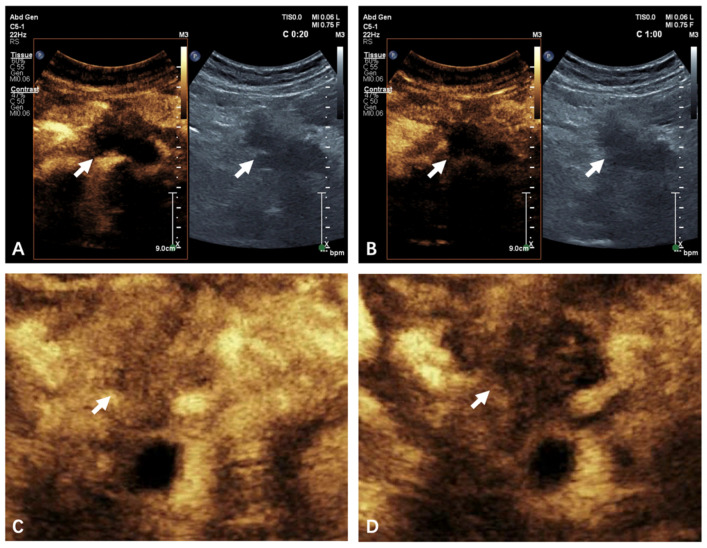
US+CEUS and CEUS-ROI images. (A,B) An example of typical US+CEUS frames of 20 s (arterial phase) (A) and 60 s (venous phase) (B) of a patient with ineffective treatment outcome, showing hypo-enhancement pattern. (C,D) An example of typical CEUS-ROI frame of 20 s (arterial phase) (C) and 60 s (venous phase) (D) of a patient with effective treatment outcome, showing iso-enhancement pattern. The white arrow indicates pancreas cancer. US: ultrasound; CEUS: contrast-enhanced ultrasound; ROI: region of interest.
